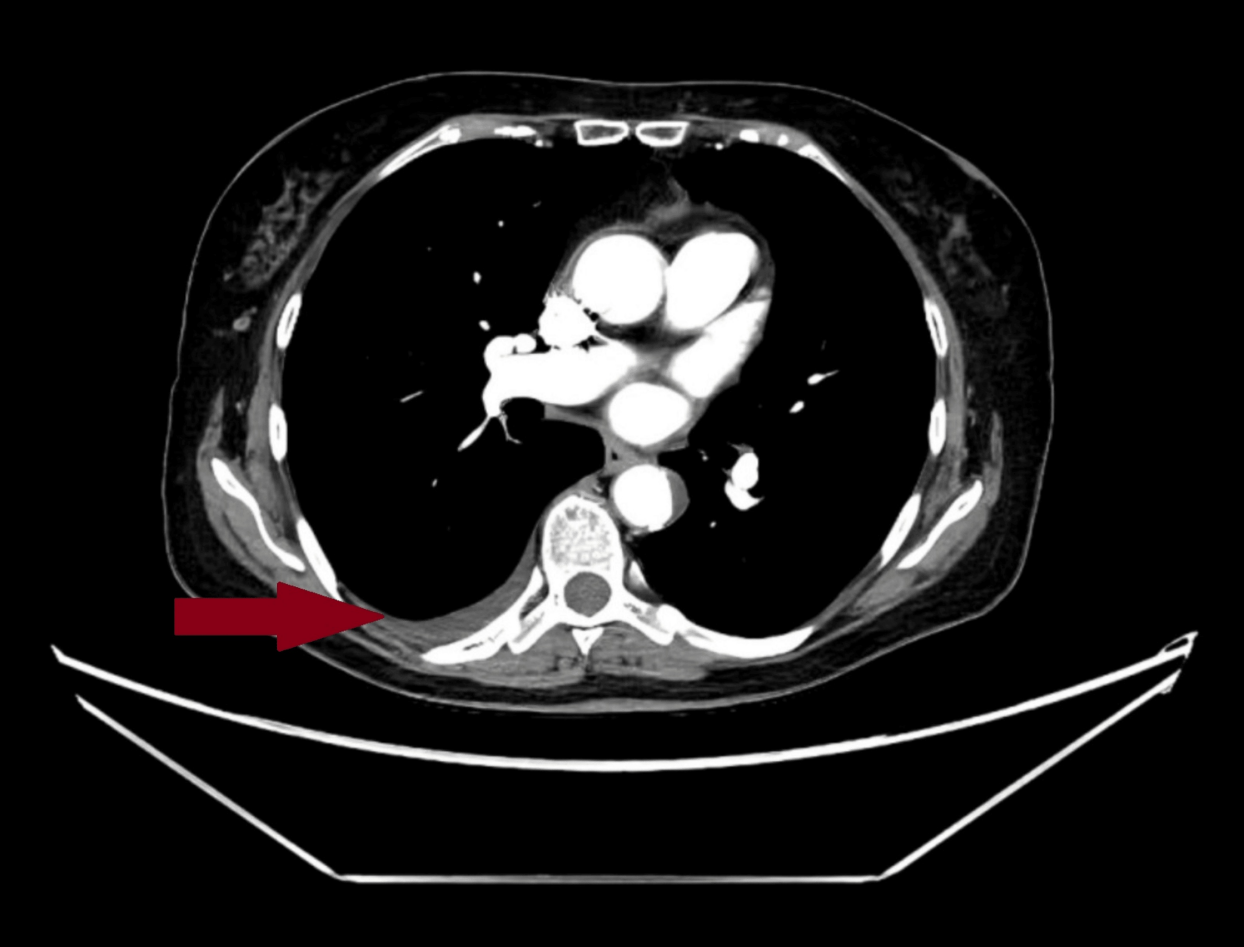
CT at discharge Visible resolution of pericardial effusion and fluid in the left pleural cavity. 7 mm of fluid in the right pleural cavity is seen. CT: computed tomography
